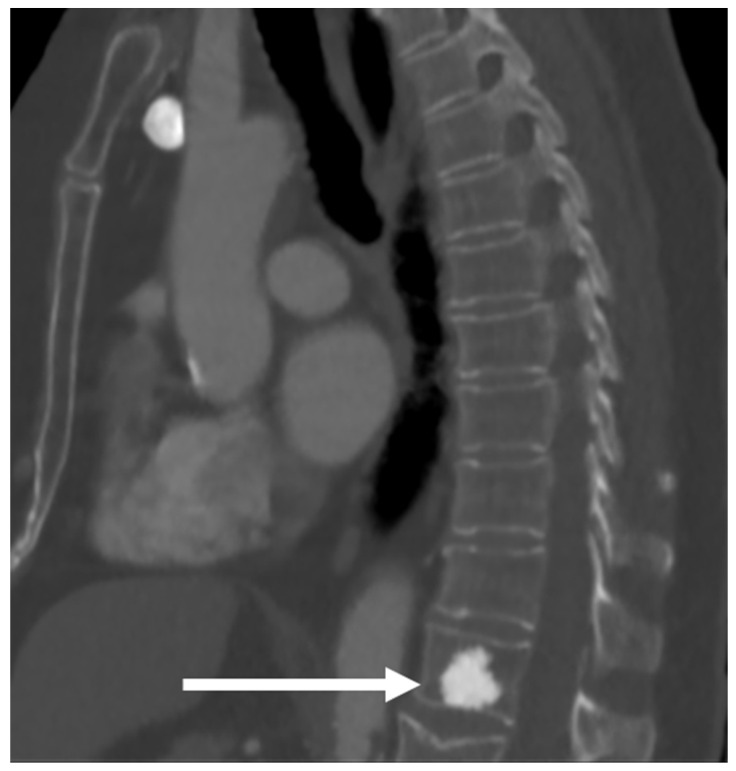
Sagittal CT demonstrating an osteoma of T12 vertebra (white arrow). Note the typical sclerotic, hyperdense appearance with spiculated margins.
